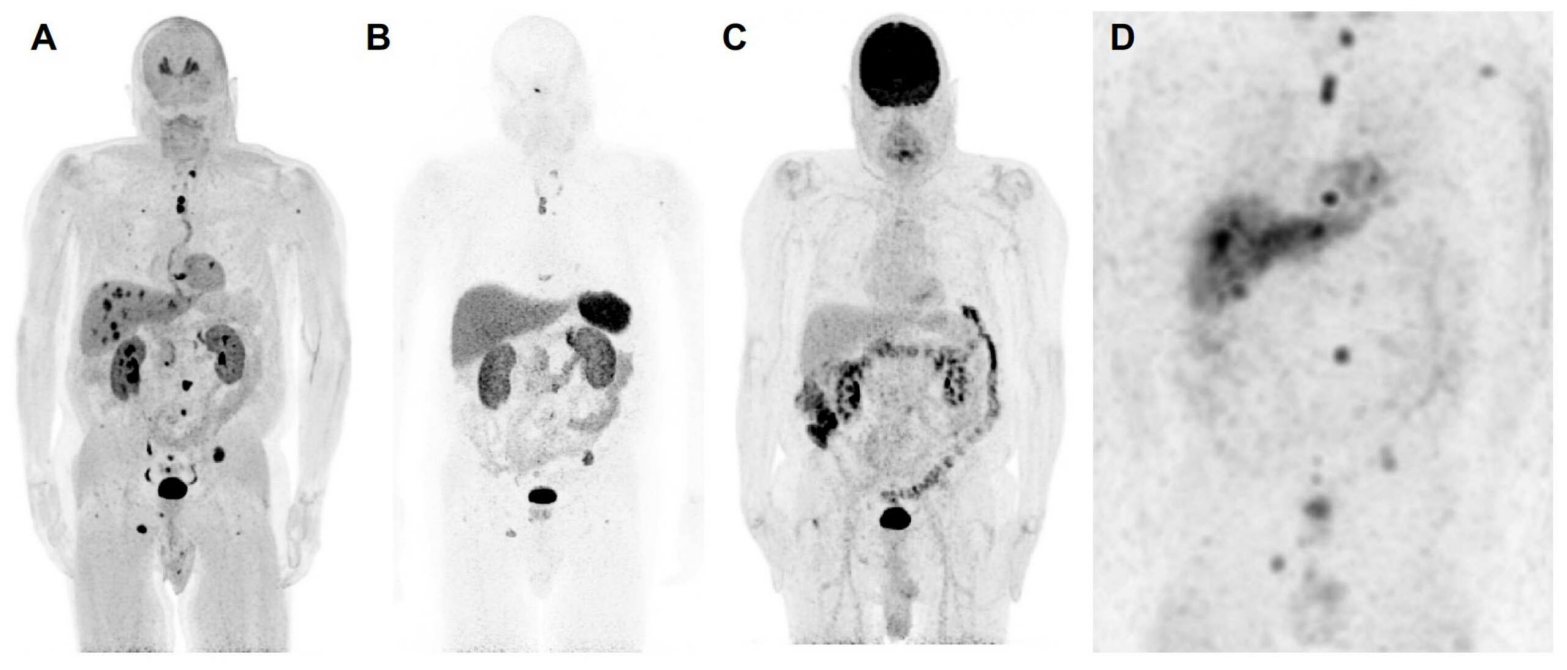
The anterior maximum intensity projection images of ^18^F-FDOPA positron emission tomography/computed tomography (PET/CT) (A), ^68^Ga-DOTATATE PET/CT (B), ^18^F-FDG PET/CT (C), and ^123^I-MIBG single photon emission computed tomography/computed tomography (SPECT/CT) (D) of a 67-year-old male (patient 3) with a likely oncogenic somatic *ATRX* variant (c.2018dup, p.Thr674fs) with a history of a previously resected 8.7 cm right pheochromocytoma demonstrates metastatic lesions in lungs, liver, and bones. ^18^F-FDOPA PET/CT shows superiority in detection of metastatic lesions compared to ^68^Ga-DOTATATE PET/CT, ^18^F-FDG PET/CT, and ^123^I-MIBG SPECT/CT. Liver lesions are detected by ^18^F-FDOPA PET/CT and ^123^I-MIBG SPECT/CT and are not seen on ^68^Ga-DOTATATE PET/CT and ^18^F-FDG PET/CT. Furthermore, ^18^F-FDOPA PET/CT detects more bone and lung lesions compared to ^68^Ga-DOTATATE PET/CT, ^18^F-FDG PET/CT, and ^123^I-MIBG SPECT scintigraphy. Based on widespread avidity of metastatic lesions on ^123^I-MIBG scintigraphy compared to ^68^Ga-DOTATATE PET/CT, the patient was recommended for high-specific-activity ^131^I-MIBG (Azedra^®^) targeted radiotherapy. Additionally, ^18^F-FDG PET/CT demonstrates diffuse increased uptake in the colon, likely physiologic and not suggestive of malignancy. The ^18^F-FDA PET/CT, unfortunately, was not performed in this patient. To note, contrast recovery, sensitivity, and spatial resolution of PET/CT imaging is superior to SPECT/CT imaging, and therefore, smaller lesions on PET/CT scans may not be visible on SPECT/CT scans despite adequate uptake.
